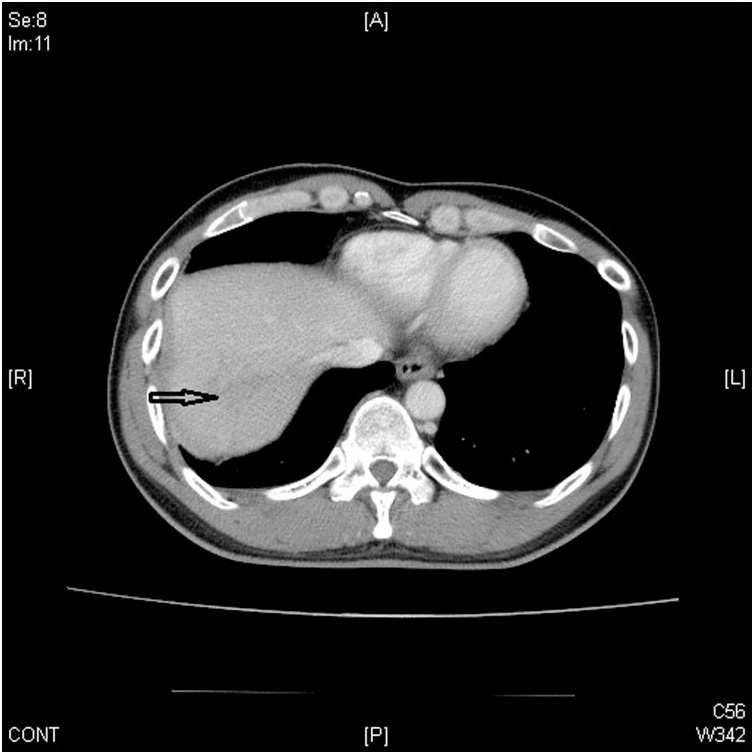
Abdominal computed tomography scan of the same patient performed 4 months after surgery, showing a well-healed liver injury and no residual abscess (arrow).
